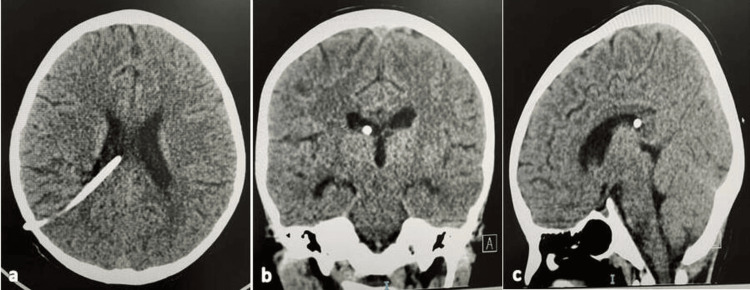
Brain CT images of an eight-year-old boy with Lhermitte-Duclos disease (LDD) after right-side ventriculoperitoneal shunt insertion (a) Axial view; (b) Coronal view; (c) Sagittal view
